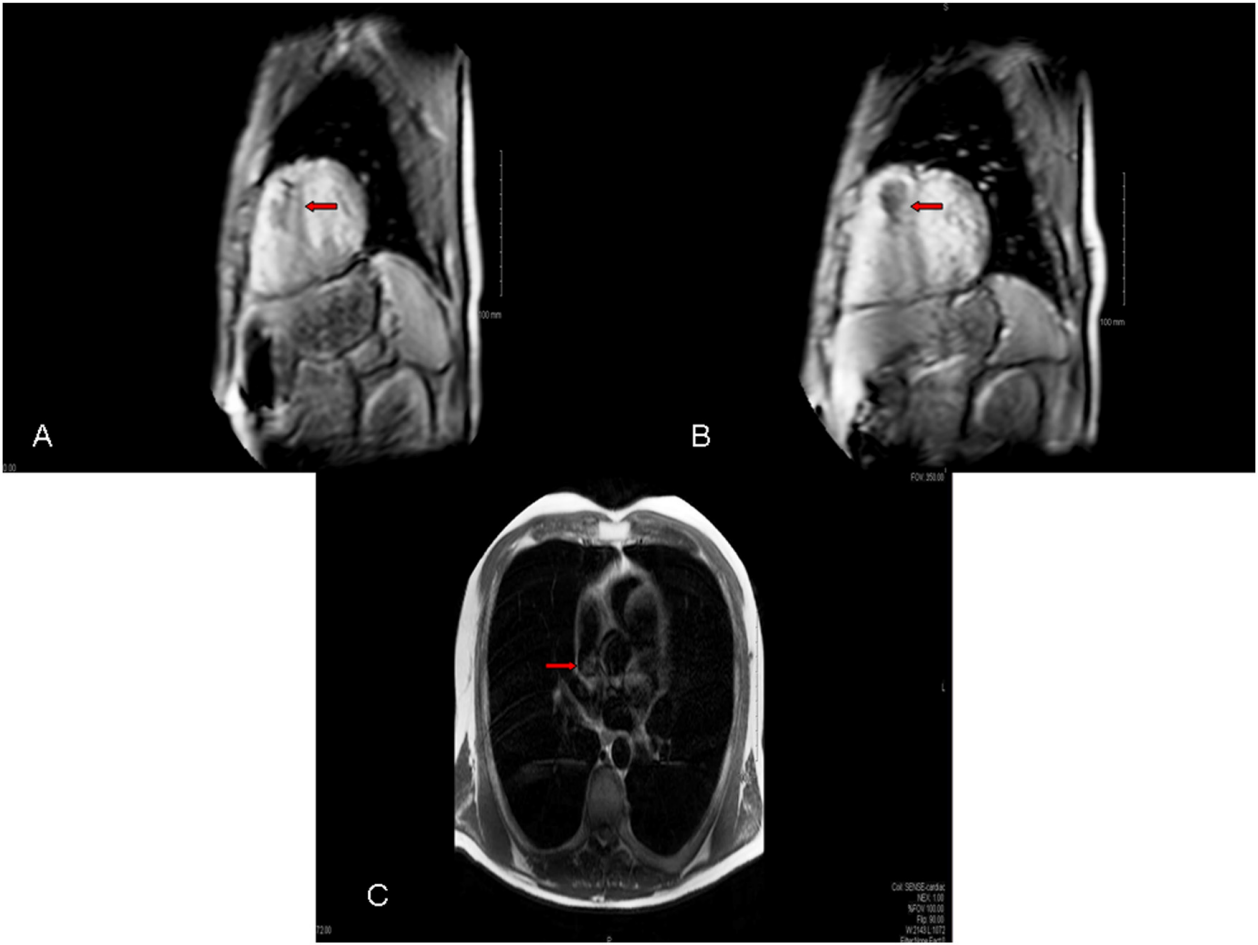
Magnetic resonance imaging. (A, B) Magnetic resonance imaging perfusion imaging showing right ventricular mass extending from the base of the right ventricle to the apex (red arrow). C: Magnetic resonance imaging pre-scan (pre-perfusion) images showing right ventricular mass (red arrow).
